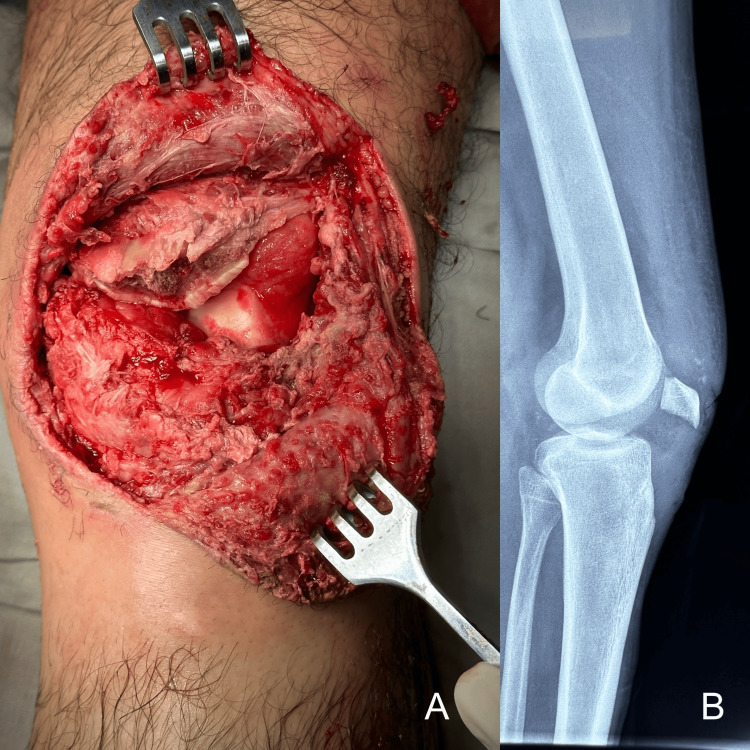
Intraoperative photographs and radiographs obtained during the second surgical debridement. (A) Intraoperative view after aggressive debridement and excision of infected soft tissue.
